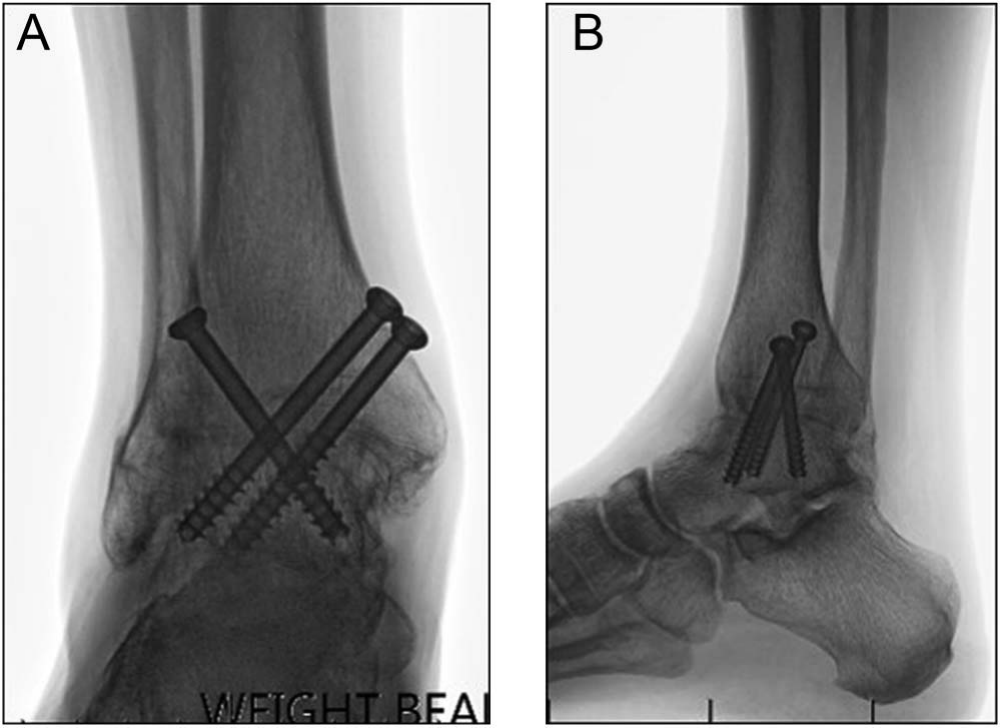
Radiographs taken 12 months after arthroscopic ankle arthrodesis (AAA) in a 55-year-old man: (A) anteroposterior view; (B) lateral view.
